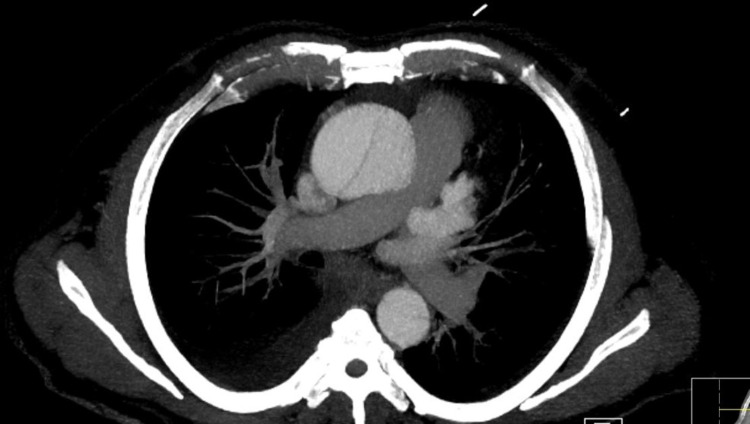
CT angiogram demonstrating descending aorta double lumen
